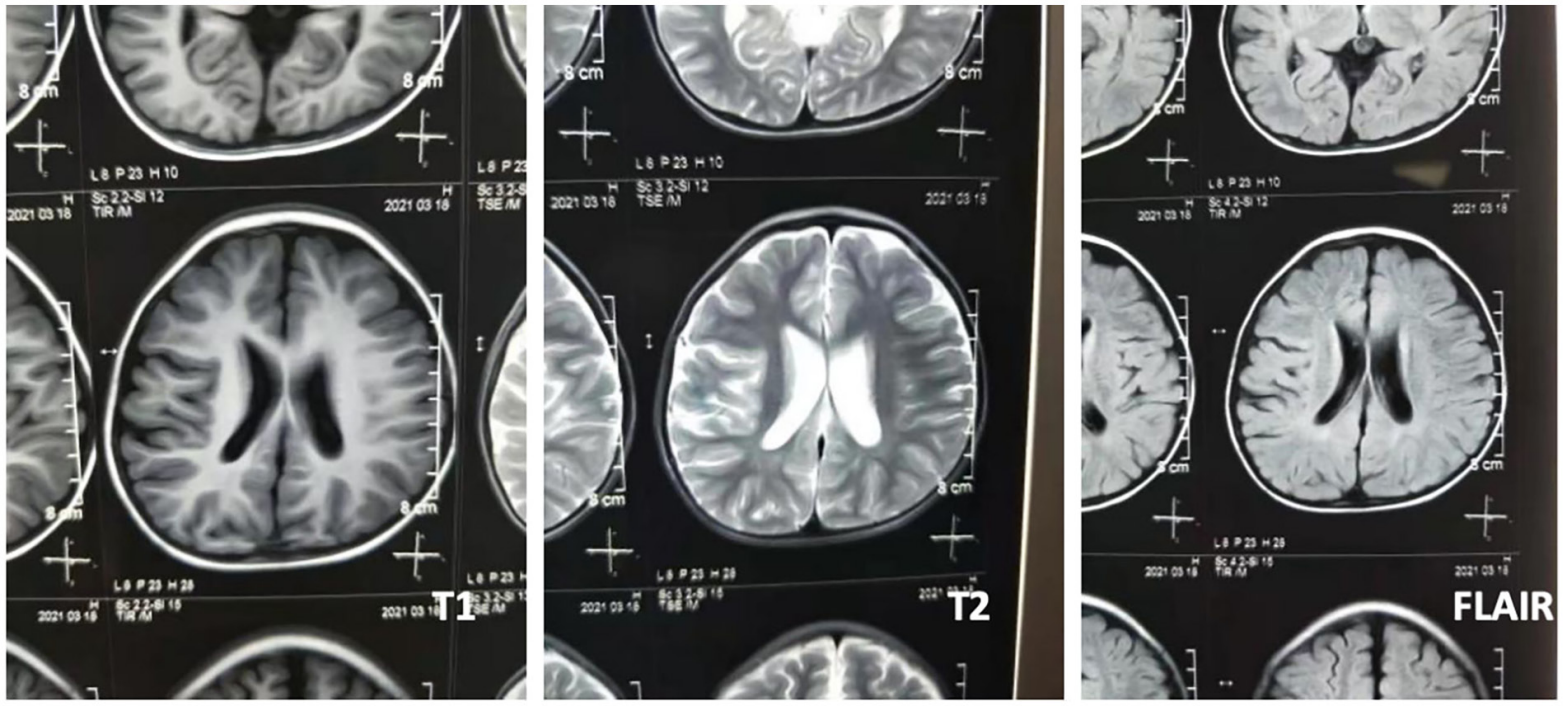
Brain MRI examination in the patient with EVEN‐plus syndrome. Features include: irregular shape of bilateral ventricles, reduction of periventricular white matter, spots of slightly longer T1 and longer T2 signal shadows in bilateral basal ganglia, FLAIR sequence showing high signal, suggesting periventricular white matter softening
